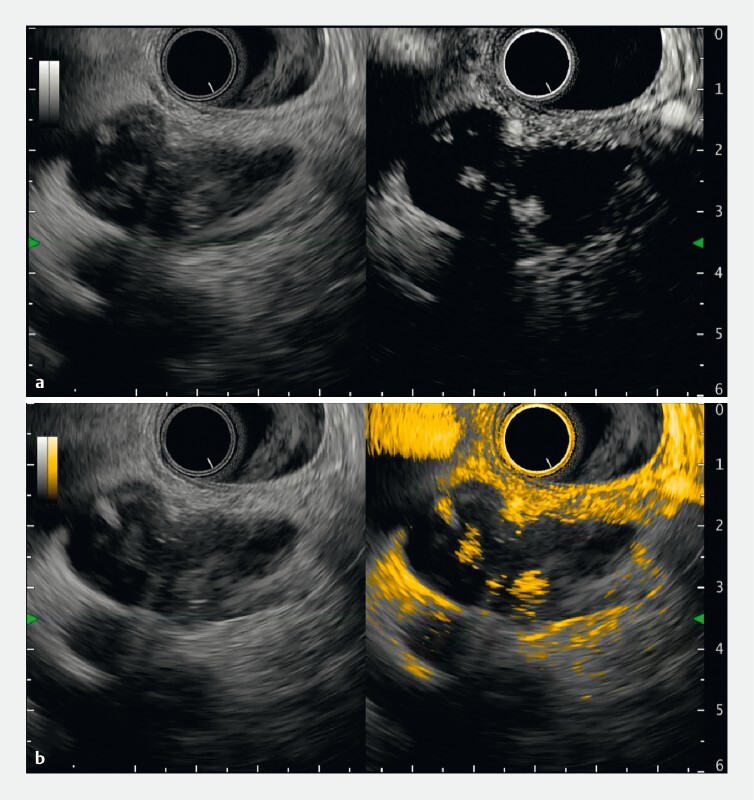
Case 1: Contrast-enhanced endoscopic ultrasound indicated enhanced mural nodules in the branch duct intraductal papillary mucinous neoplasm.
